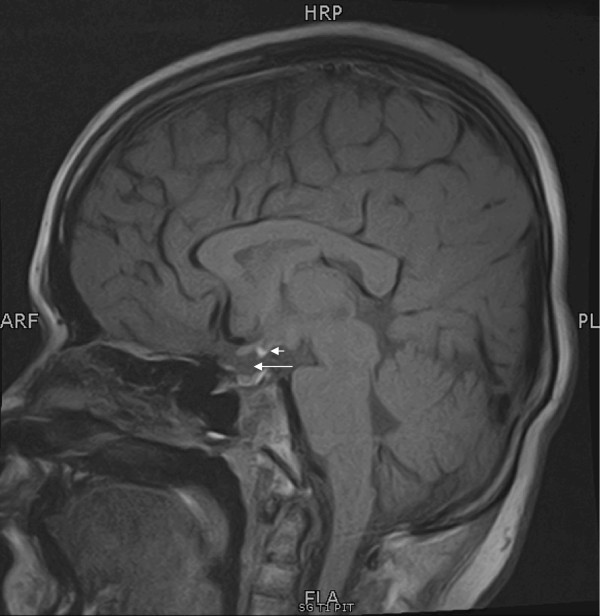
T1 weighted magnetic resonance image of the pituitary. Pituitary stalk is absent (long arrow). Posterior pituitary is not seen in relation to the anterior pituitary in the pituitary fossa and is seen as hyperintense focus at the median eminence (short arrow). Anterior pituitary is seen in pituitary fossa at its normal location and returning a normal signal.
